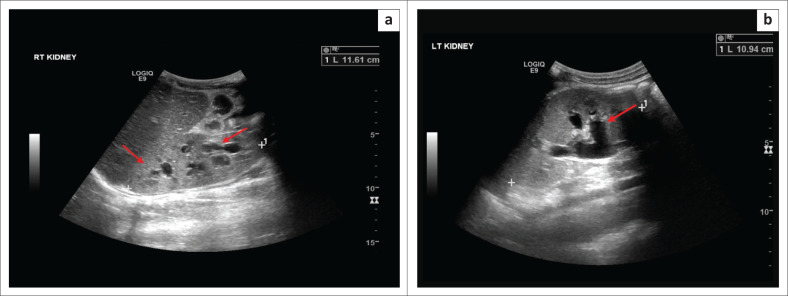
Ultrasound of the right kidney (a) and left kidney (b) demonstrating bilateral hydronephrosis and enlarged kidneys.
